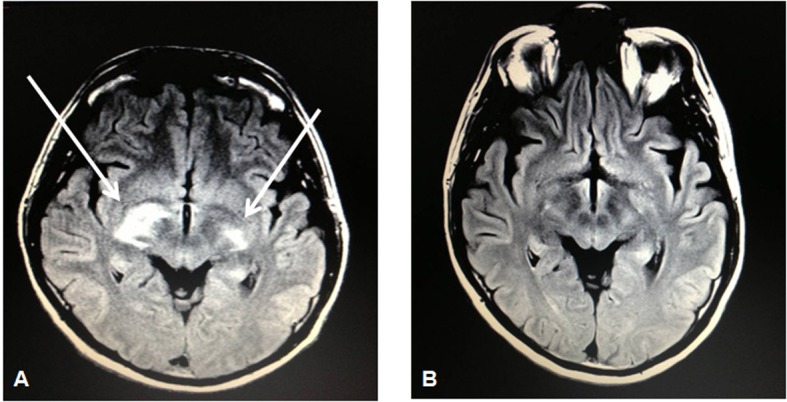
MRI brain showing bilateral signal enhancement (more on the right than left side) within the lateral subthalamus, midbrain, and internal capsule (A) followed by resolution of such lesions on a repeat MRI brain 5 months later (B) after 3 cycles of intravenous pemetrexed 900 mg/m^2^.
